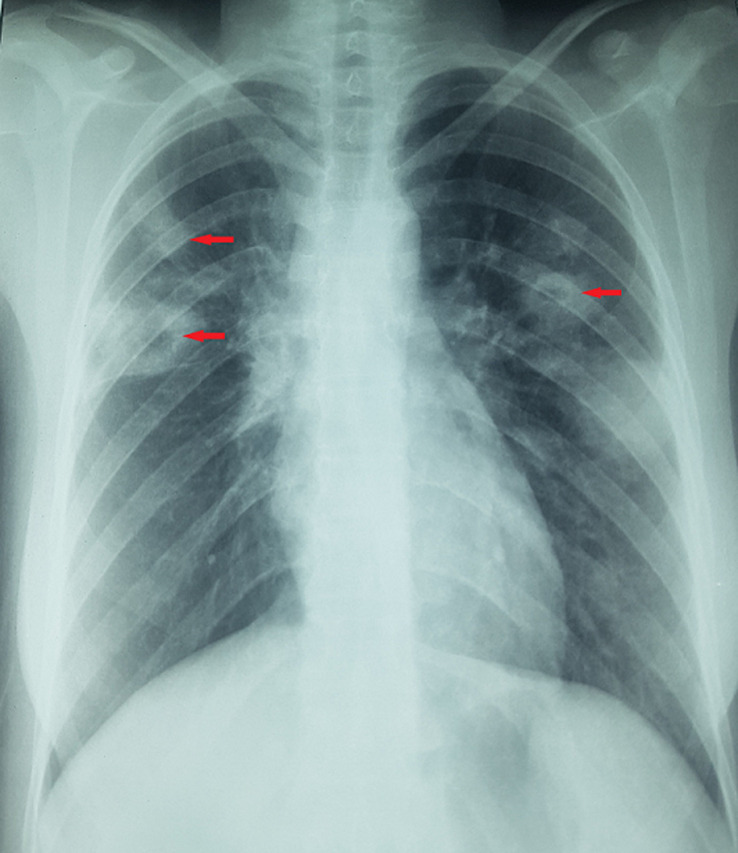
chest X-ray showing bilateral excavated opacities in the upper lungs
